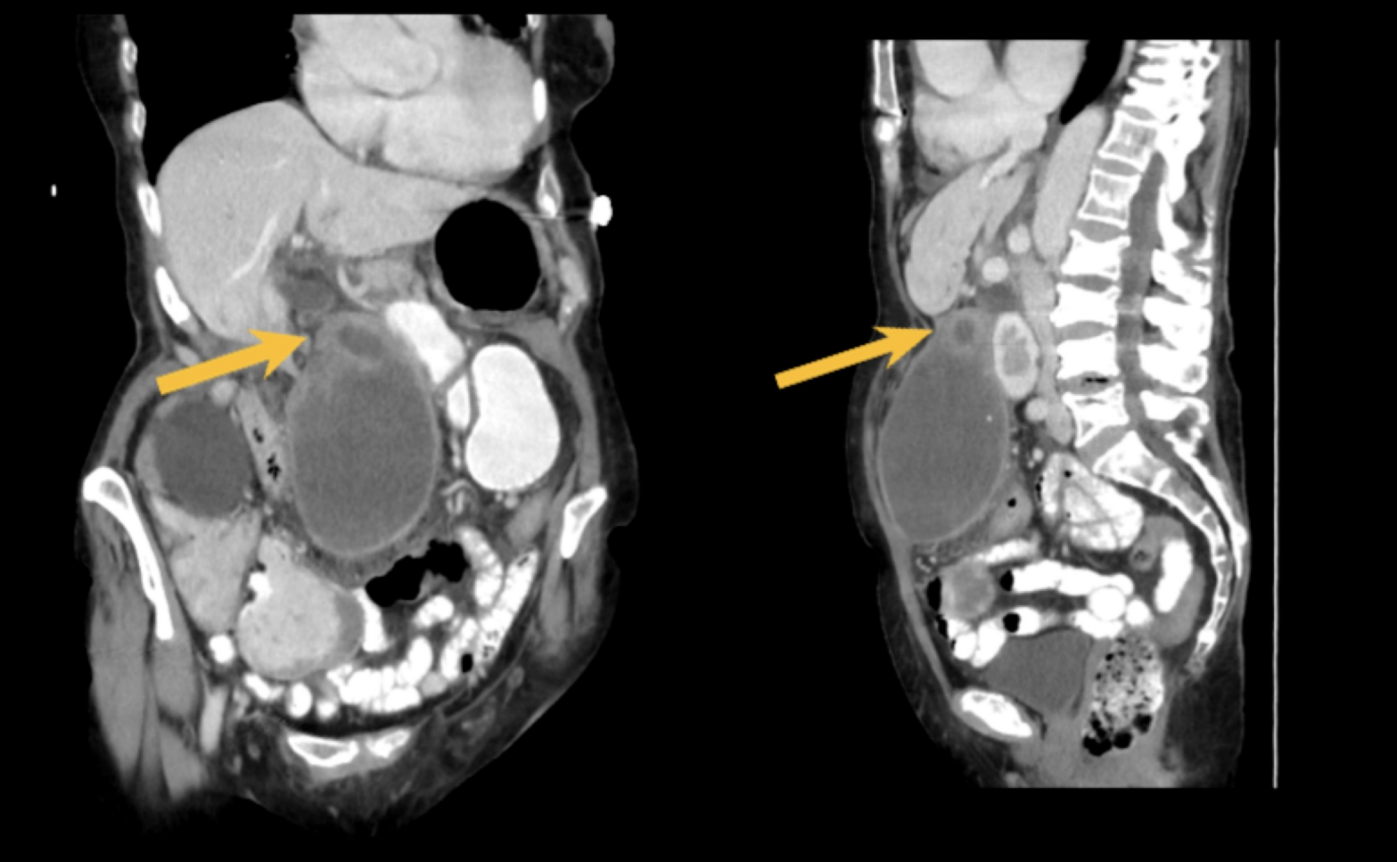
Left image: coronal section CT abdomen/pelvis with IV contrast, with the yellow arrow demonstrating a possible sight of gallbladder perforation/discontinuity; Right image: sagittal section of CT abdomen/pelvis with IV contrast, with the yellow arrow demonstrating possible perforation/discontinuity. CT = computed tomography; IV = intravenous
